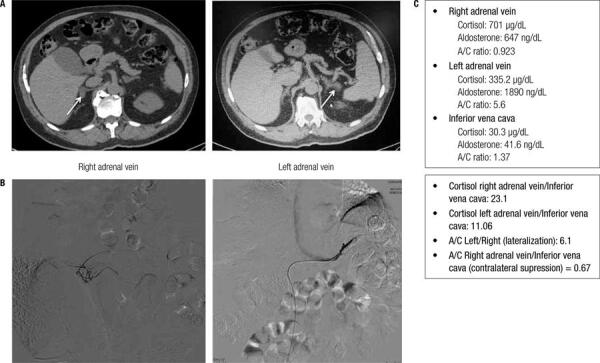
A male patient, 69 years, with resistent hypertension for 30 years, diagnosed with PA. Hormonal data: aldosterone (A) = 24.4 ng/dL; DRC < 1.6 mU/mL (PRA 0.13); A/DRC ratio = 15.25; A/PRA ratio = 188. (A) Adrenal CT showing a 1.8 cm nodule at right adrenal gland and a 1.2 cm nodule at left adrenal gland. (B) Fluoroscopic imaging from AVS. (C) Analysis of AVS sampling showed lateralization of aldosterone production to the left side. Then, this patient had an adrenal incidentaloma on the right side and an aldosterone-producing adenoma on the left side. The patient underwent left adrenal adrenalectomy and had biochemical cure of PA.
